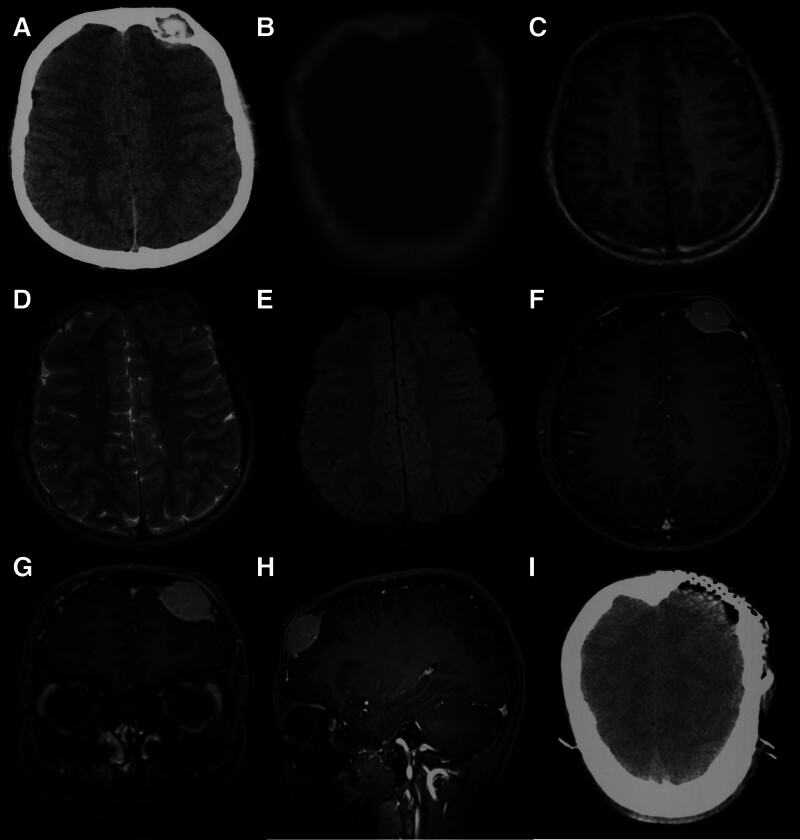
Preoperative images of computed tomography (CT) scans, magnetic resonance imaging (MRI) and postoperative images of CT. (A) CT scan of the head showing a round-shaped high and low-density shadow in the left side of the frontal bone. (B) CT bone window showing the mass penetrating the skull and invading the intracranial. (C–E) The signal is slightly lower and higher on T1WI, T2WI, and DWI. (F–H) Post-contrast MRI showing a markedly enhanced mass in the left frontal bone, along with enhancement of the adjacent meninges. (I) Postoperative CT image showing complete removal of the lesion.
